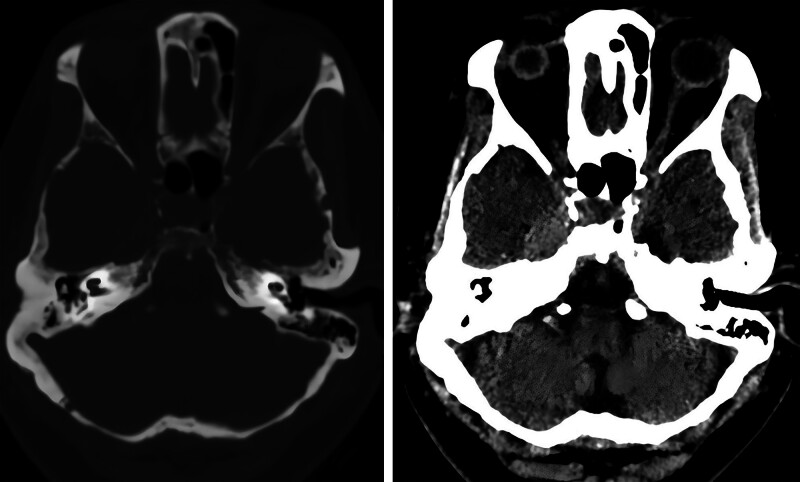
CT imaging shows no evidence of calcification, hemorrhage, necrosis, or significant bony hyperostosis/destruction within detectable limits. CT = computed tomography.
